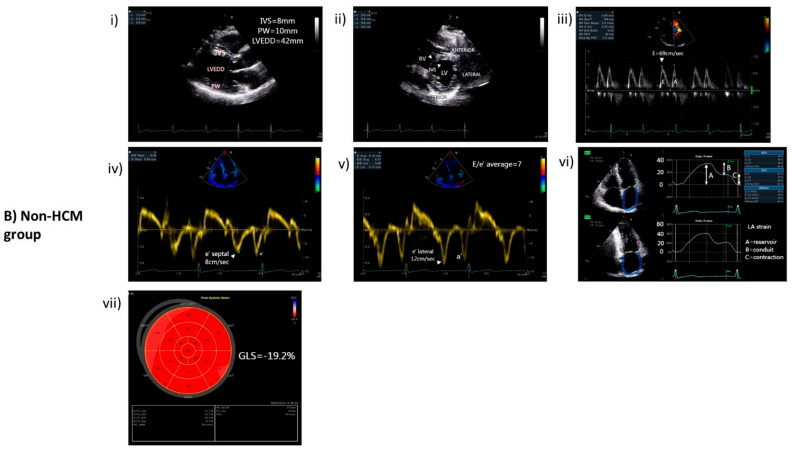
Representative echocardiographic images from HCM and non-HCM groups. (A) (i–ix) images from a patient with obstructive HCM; (i) 2D PLAX view at end-diastole, (ii) 2D PLAX view at end-systole, (iii) M-Mode through the LVOT, (iv) CW Doppler through LVOT, (v) PW Doppler of mitral inflow, (vi–vii) Doppler tissue imaging velocities of the medial and lateral mitral annulus, respectively; (viii) LA strain, (ix) LV GLS; Note the asymmetric hypertrophy of anterior IVS, the dilated anteroposterior diameter of LA, the SAM of the mitral valve that causes LVOT obstruction with a maximum PG of 93 mmHg at rest, and the diastolic dysfunction with a restrictive mitral inflow pattern and low diastolic tissue velocities. Moreover, observe the low absolute values of the LA strain and LV GLS. (A) (x–xiv) images from a patient with non-obstructive HCM; (x) 2D PLAX view at end-diastole, (xi) 2D SAX view at the level of papillary muscles at end-diastole, (xii) M-Mode through LVOT, (xiii) LA strain, (xiv) LV GLS, Note the marked thickening of the anterior IVS, the absence of SAM of the mitral valve and LVOT obstruction at rest or after a Valsalva maneuver. Furthermore, observe the low absolute values of the LA strain and LV GLS. (B) (i–vii) images from a patient without HCM; (i) 2D PLAX view at end-diastole, (ii) 2D SAX view at the level of papillary muscles upon end-diastole, (iii) PW Doppler of mitral inflow, (iv-v) Doppler tissue imaging velocities of the medial and lateral mitral annulus, respectively; (vi) LA strain, (vii) LV GLS. Note the normal shape and thickness of LV, the normal mitral inflow pattern and LV tissue velocities. Observe the normal absolute values of LA strain and LV GLS. Abbreviations: HCM: hypertrophic cardiomyopathy; 2D: two-dimensional ultrasound; PLAX: parasternal long-axis view; LVOT: left ventricular outflow tract; CW: continuous-wave Doppler; PW: pulsed-wave Doppler; PG: peak gradient; LA: left atrium; LV: left ventricle; SAM: systolic anterior motion of mitral valve; GLS: global longitudinal strain; IVS: interventricular septum; SAX: short-axis view.
